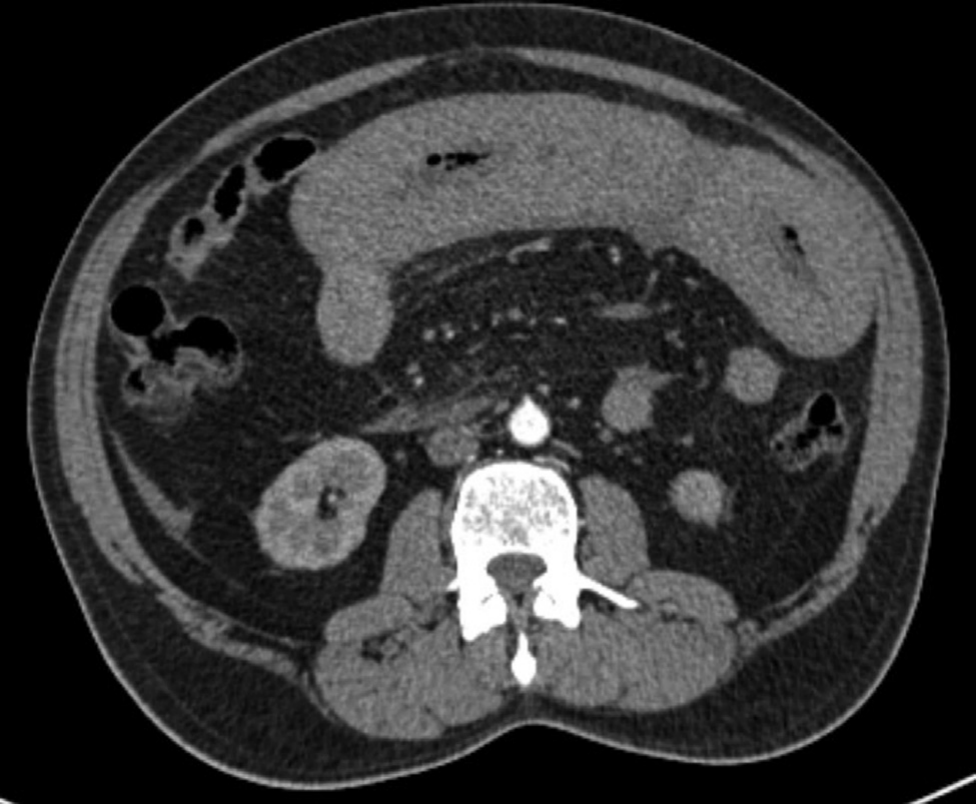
Contrast-enhanced computed tomographic scan of the abdomen showing intramural jejunal hematoma.
